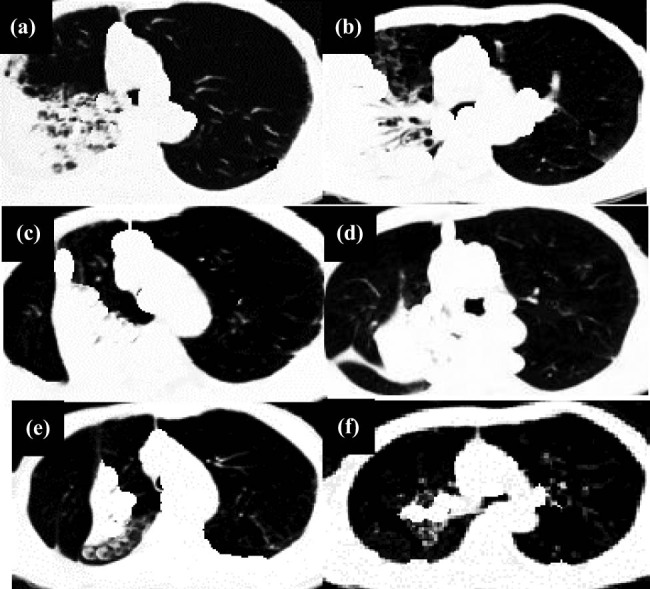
Another 55-year-old male patient with repeated cough for more than 2 years, was admitted to the hospital for recurrent chest pain for 3 days. Physical examination showed a small number of moist rales in the right upper lung. (a) and (b) initial CT images at admission, (b) and (c) initial CT images during the treatment, and (d)–(f) initial CT images 2 months after the treatment.
